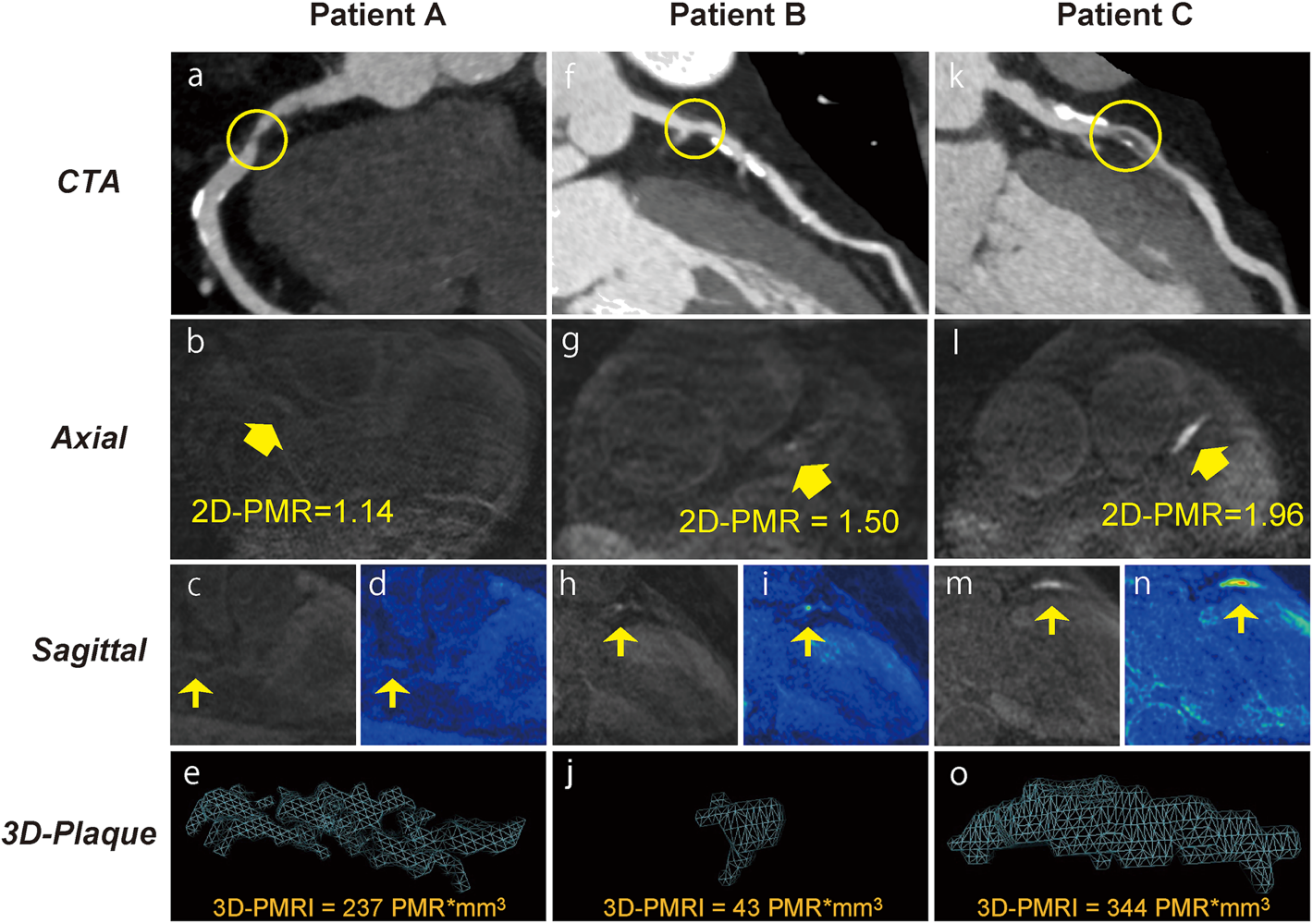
Representative 2-dimensional and 3-dimensional plaque assessment on T1-weighted imaging. Coronary plaques with 2D^low^3D^high^ in the proximal right coronary artery (2D-PMR, 1.14; 3Di-PMR, 237 PMR*mm^3^; Patient A: a–e), 2D^high^3D^low^ in the proximal left anterior descending artery (LAD) (2D-PMR, 1.50; 3Di-PMR, 43 PMR*mm^3^; Patient B: f–j), and 2D^high^3D^high^ in the proximal LAD (2D-PMR, 1.96; 3Di-PMR, 344 PMR*mm^3^; Patient C: k–o). Computed tomography angiography (CTA) images (a, f, k), and axial images (b, g, l), sagittal images (c, h, m), color maps (d, I, n), and 3D region of interests (3D plaque: e, j, n) on T1w images are shown. Yellow circles indicate percutaneous coronary intervention target lesion sites on CTA. Yellow arrows indicate lesions on T1w imaging corresponding to a lesion on angiography that underwent intervention.
